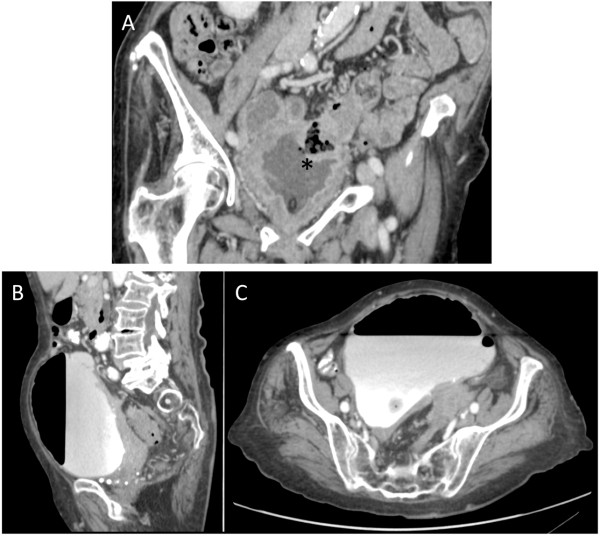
(A) Coronal reformatted CT acquired before the CTC shows an obvious colovesical fistula (asterisk).(B,C) After insertion of a Foley catheter and insufflation of air in the rectum, a great quantity of air appeared in the bladder, without colon distension
